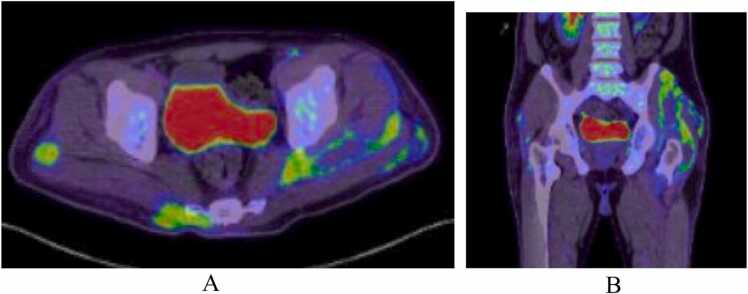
A and B PET CT FDG uptake scan demonstrates an ill-defined collection with peripheral increased FDG uptake and central necrosis, involving gluteus maximus, gluteus medius, and minimus, measuring 15 cm by 8 cm by 15 cm in maximal dimensions. There is also a focal area of fat stranding in the subcutaneous fat of the right gluteal region with peripheral FDG uptake measuring about 5 cm by 3.3 cm by 10 cm in maximal dimensions.
